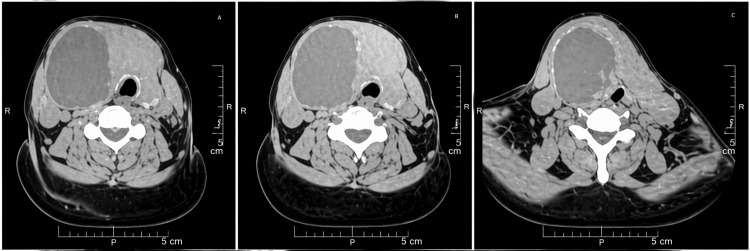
Computed tomography (CT) scan of the neck CT neck showing marked thyroid enlargement with tracheal compression and deviation, explaining the anticipated difficult airway. A: superior level showing thyroid mass compressing the trachea; B: midlevel showing enlarged thyroid with tracheal deviation; C: inferior level showing thyroid lobes and tracheal lumen narrowing.
